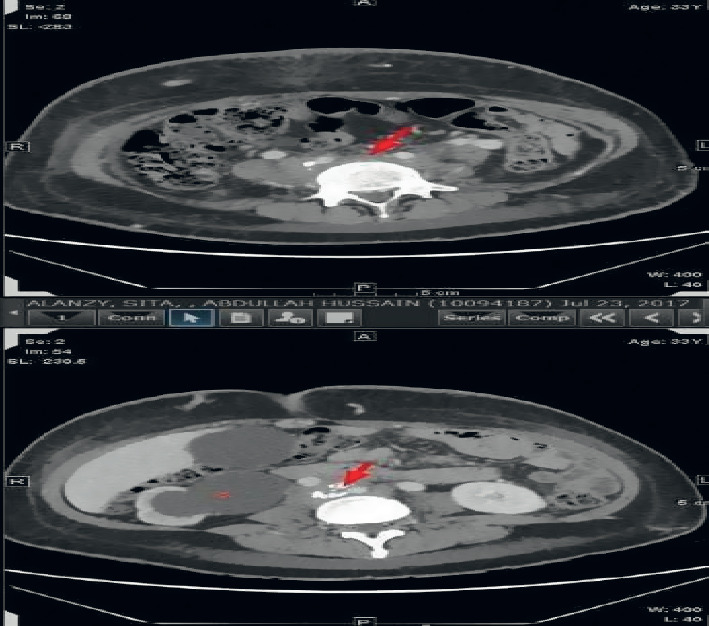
CT abdomen showing retroperitoneal fibrosis (arrow) and right hydroureter (asterisk).
